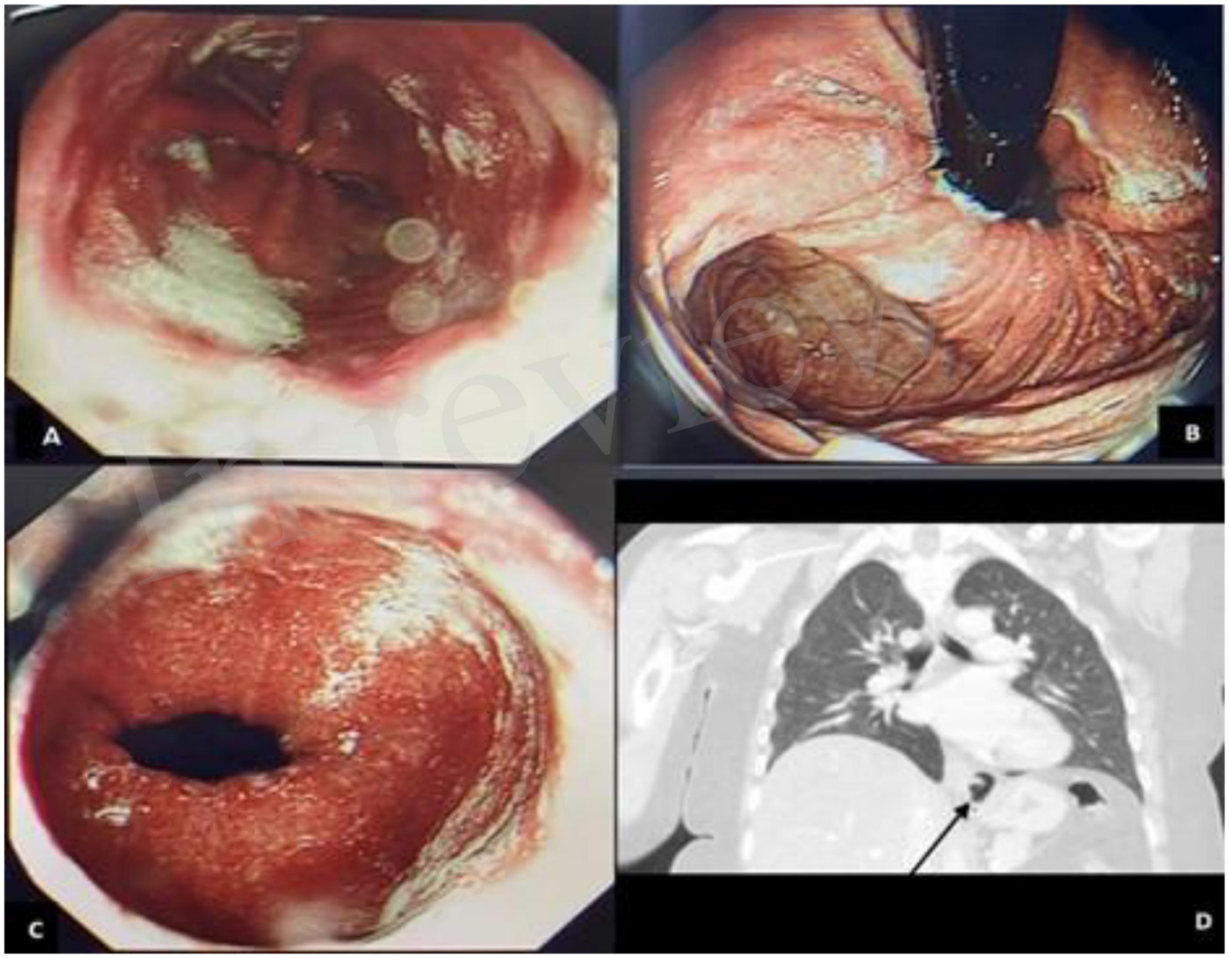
Preoperative diagnostic findings. (A) Erosions at the lower esophageal sphincter (LES) on endoscopy. (B) Endoscopic view demonstrating hiatal hernia. (C) Hyperemia of the prepyloric stomach. (D) Preoperative contrast-enhanced CT scan confirming the presence of hiatal hernia.
